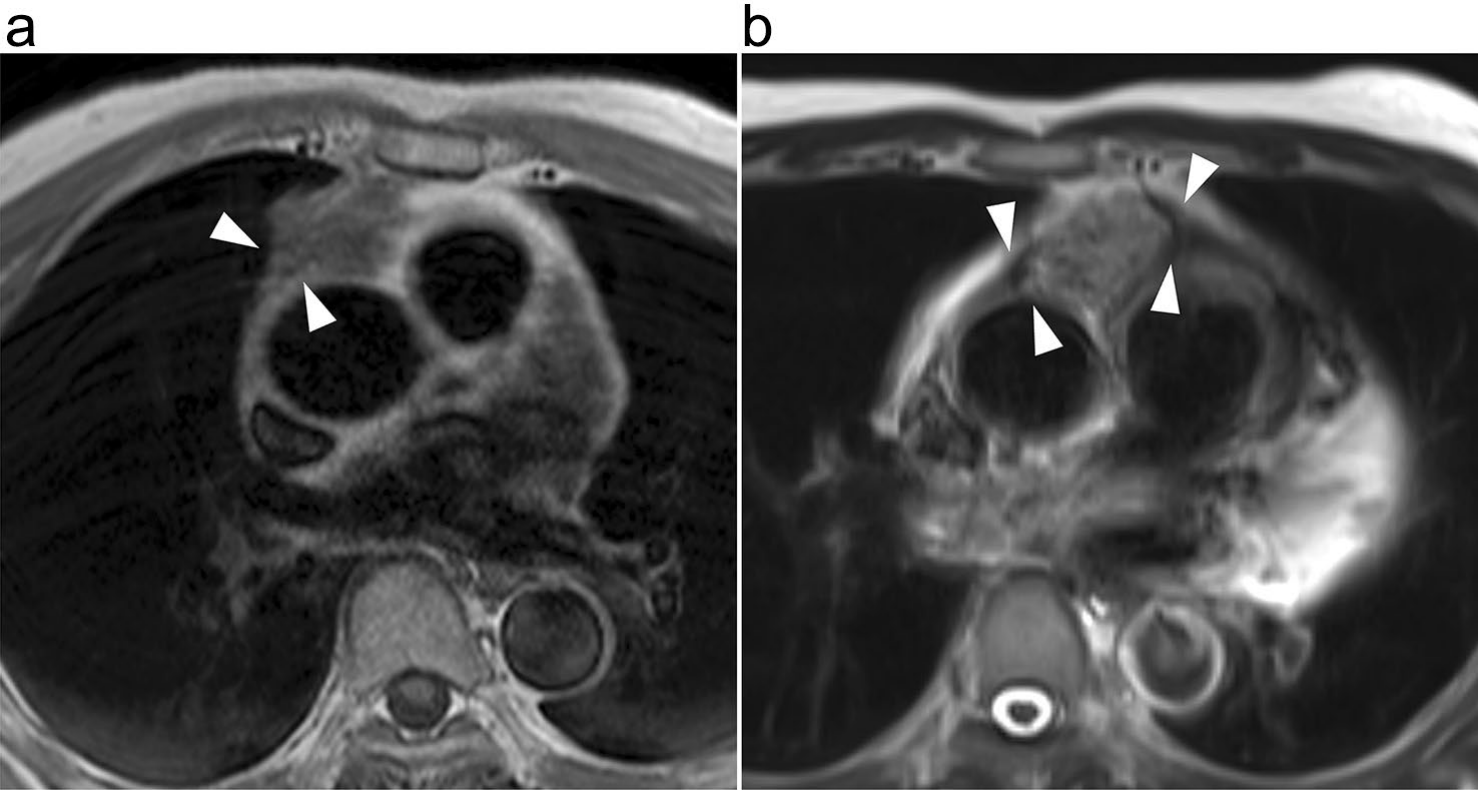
The presence of pericardial thickness/nodule in two patients. a 57-year-old man with thymic squamous cell carcinoma. T1-weighted MR image shows an anterior mediastinal tumor with thickened pericardium (arrowheads). b 63-year-old man with thymic adenocarcinoma. T2-weighted MR image shows an anterior mediastinal tumor directly in contact with and thickening the pericardium (arrowheads)
